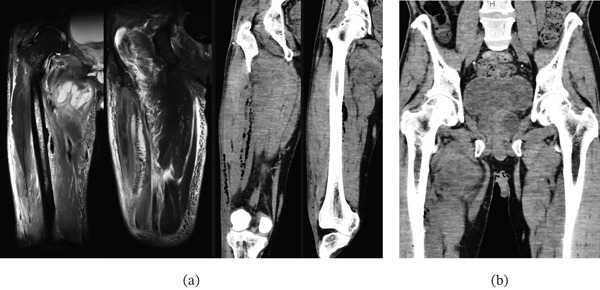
Case 2. (a) Preoperative coronal MRI with STIR image of the right hip and femur, a coronal CT image of the right hip and femur, and (b) a coronal CT image of the lower lumbar spine and hip.
